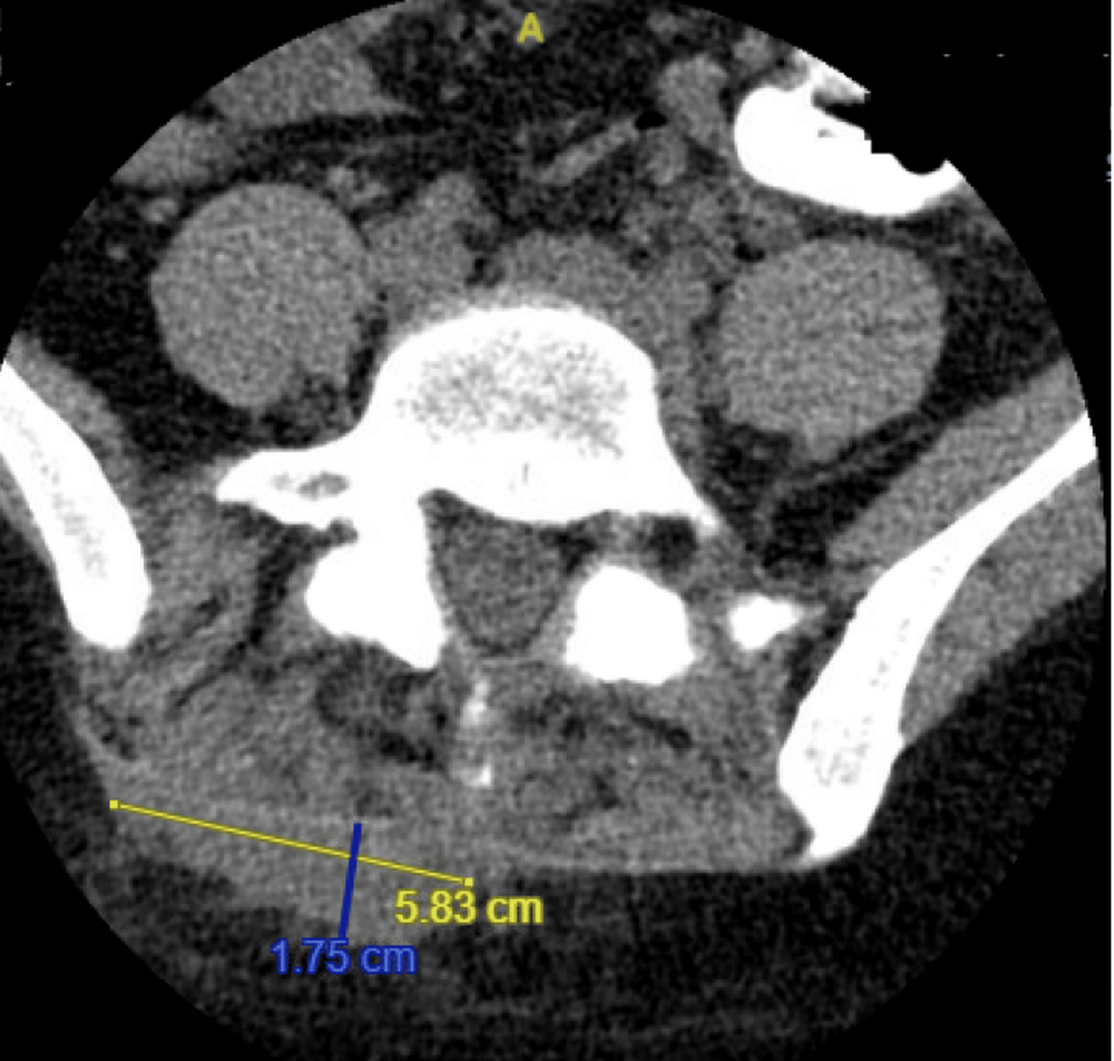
Non-contrast CT of the lumbar spine demonstrates a complex subcutaneous abscess (1.8 cm anterior x 5.8 cm transverse x 5.6 cm craniocaudal) surrounding inflammatory stranding.
